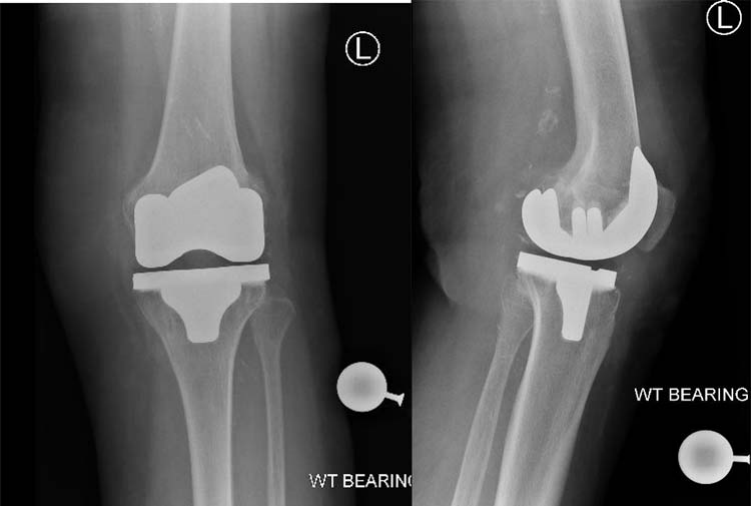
Post-operative radiographs showing satisfactory position of prosthesis for left total Knee replacement.
